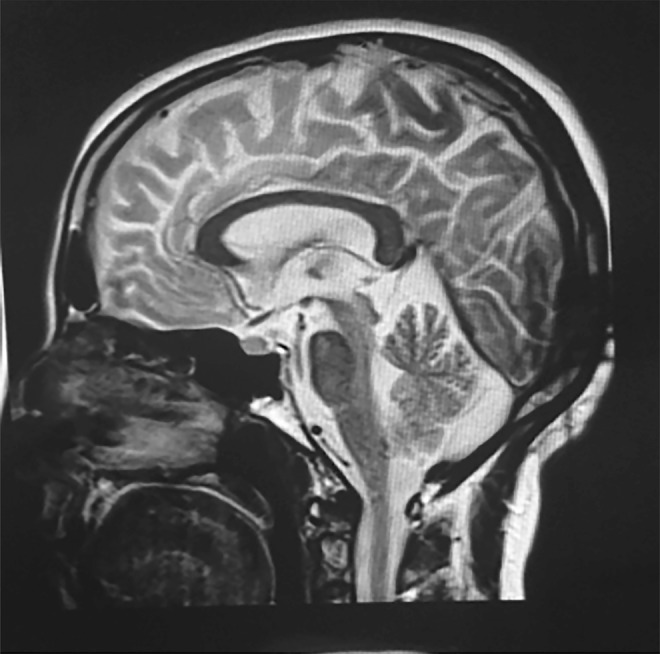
Sagittal T1-weighted MRI of brain Brain MRI from the index case (V:2) shows significant atrophy of the cerebellar vermis. The brainstem is relatively preserved.
